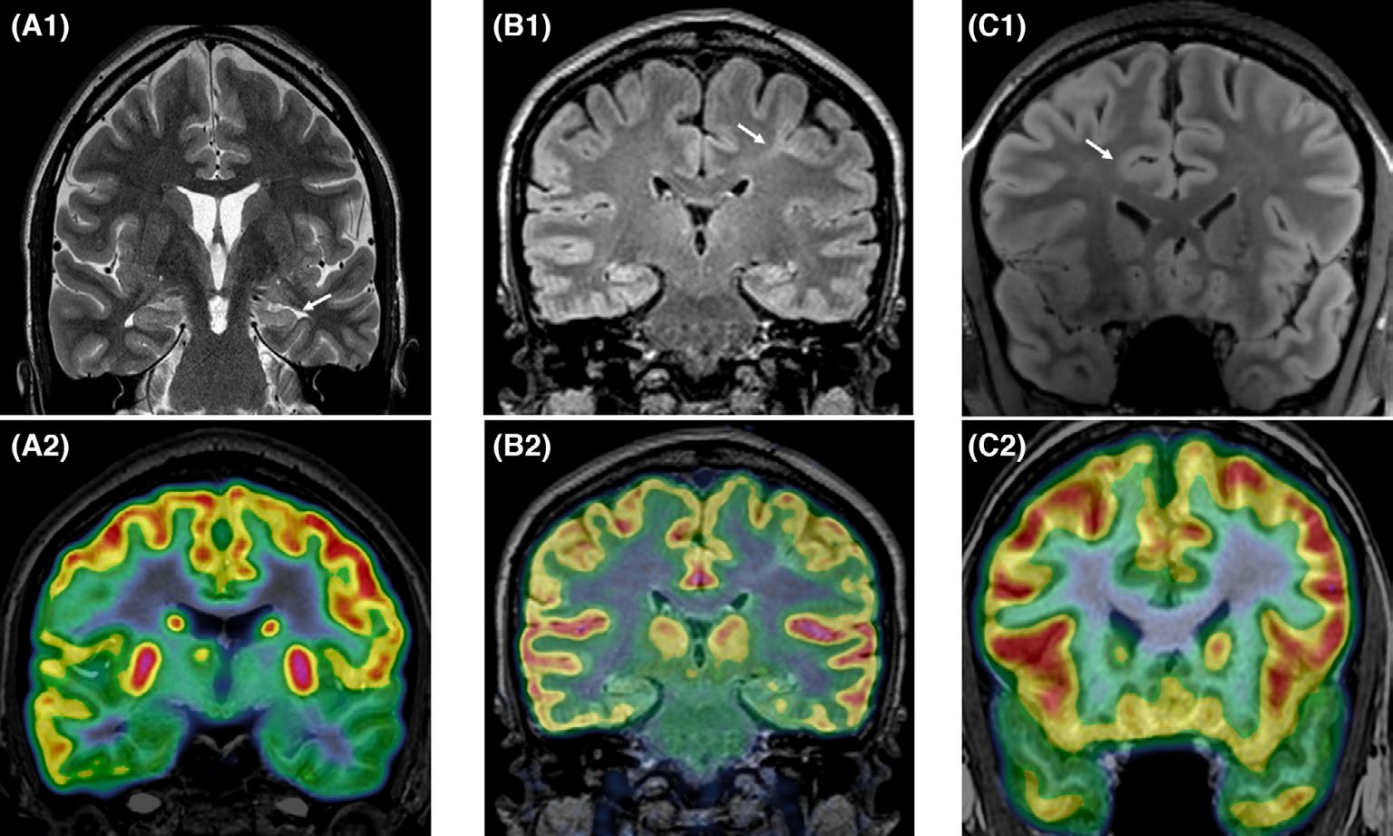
The hypometabolism involving the left temporal lobe on FDG‐PET (a2, fused 3 Tesla MRI/FDG‐PET) in a patient with hippocampal sclerosis (a1, T2‐weighted 3 Tesla MRI, indicated by arrow). Focal bottom of sulcus FDG‐PET hypometabolism (b2, fused 3 Tesla MRI/FDG‐PET) in a patient with focal cortical dysplasia with a typical transmantle sign (b1, FLAIR weighted 3 Tesla MRI) in the left premotor area. Subtle focal cortical dysplasia in the right cingulate gyrus (c1, FLAIR weighted 3 Tesla MRI) with a focal FDG‐PET hypometabolism (c2, fused 3 Tesla MRI/FDG‐PET).
